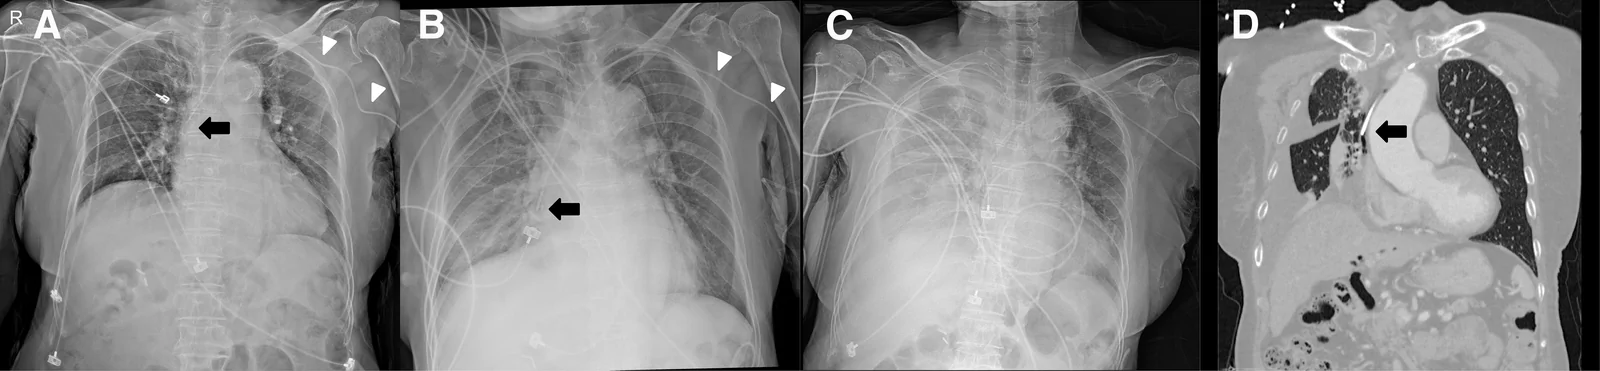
Serial chest radiographs and coronal CT image showing PICC progression and complications. (A) Chest radiograph taken immediately after peripheral central catheter insertion. The black arrow indicates the catheter tip at the midlevel of the fifth thoracic vertebra. White arrowheads mark the proximal portion of the catheter, which shows subtle tortuosity. The subtle tortuosity, considered together with the oblique orientation of the catheter tip relative to the expected central venous axis, was retrospectively interpreted as a potential sign of mechanical tension. (B) Chest radiograph obtained on postprocedural day 1. The catheter tip (black arrow) migrated caudally to the sixth thoracic vertebra. The previously observed tortuosity of the proximal catheter (white arrowheads) resolved, suggesting that this tension was released as the tip migrated caudally. (C) Chest radiograph on postprocedural day 5 demonstrating nearly total opacification of the right hemithorax, consistent with massive pleural effusion. (D) Coronal contrast-enhanced chest CT demonstrating extravascular migration of the catheter (black arrow) from the left innominate vein into the mediastinum. CT = computed tomography, PICC = peripherally inserted central catheter.
